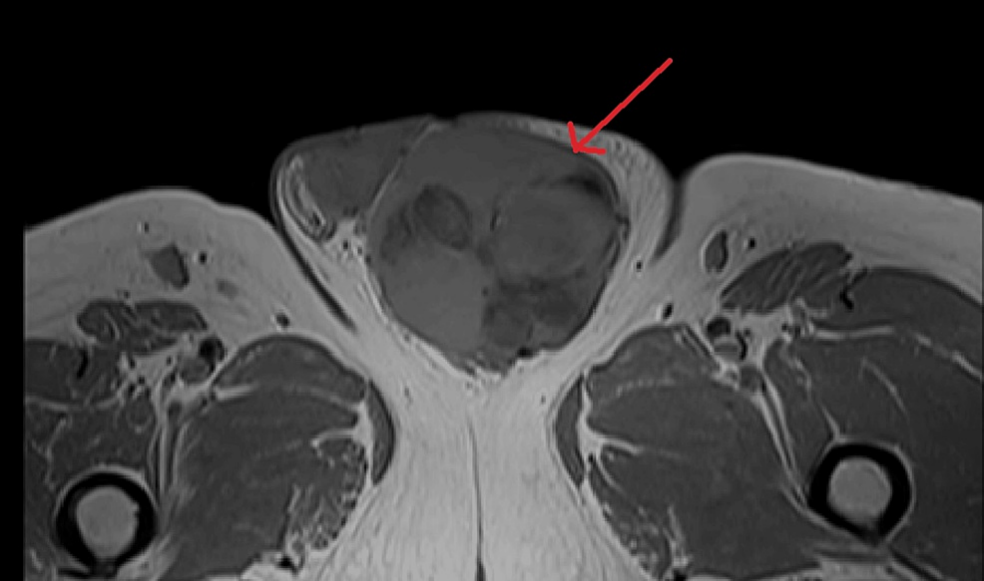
MRI T1 axial section show a multilobulated lesion with heterogenous signal in the left para testicular region.
